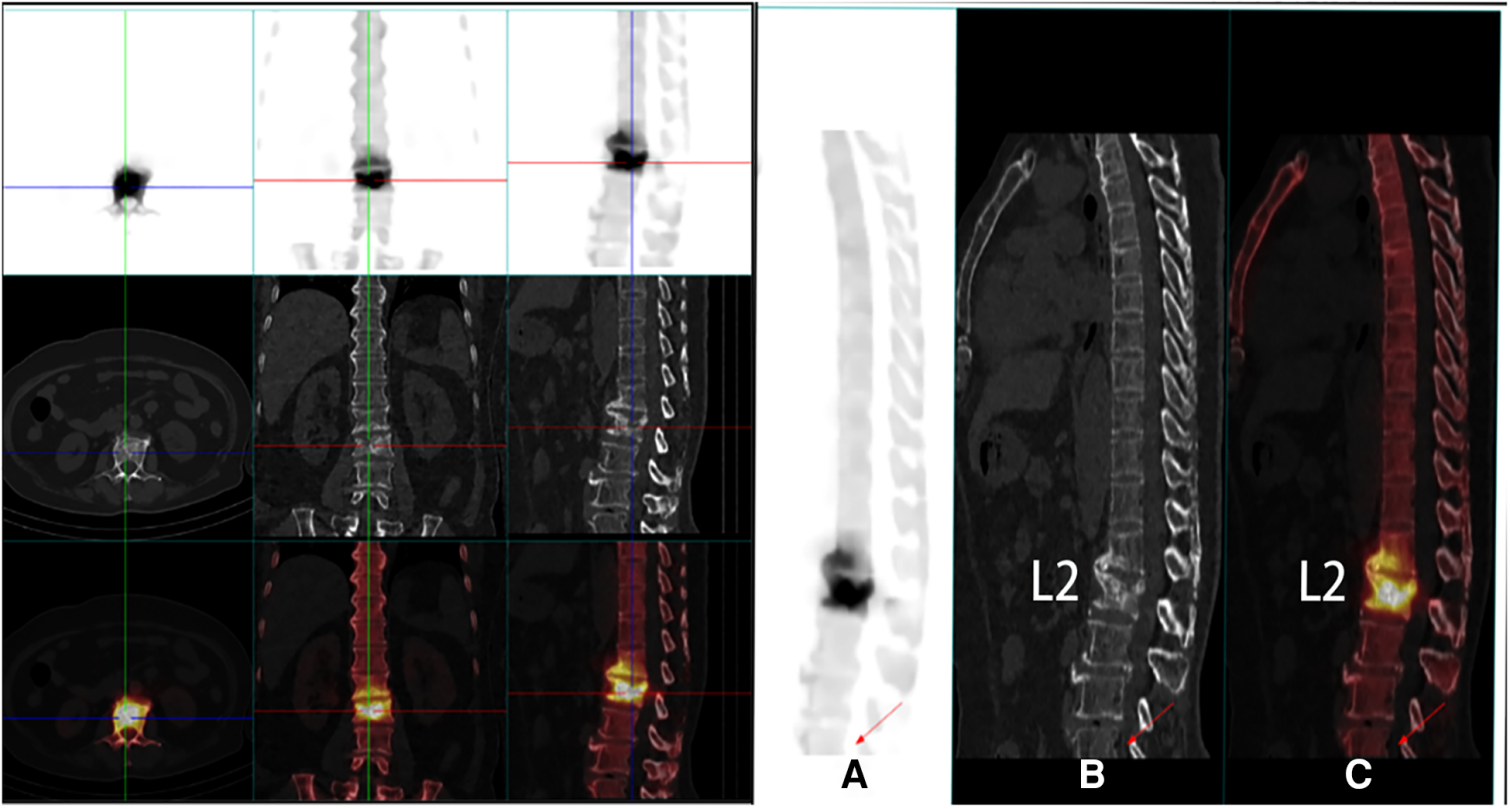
PET-CT images of the patient's preoperative coronal and sagittal vertebral fractures (A–C).
